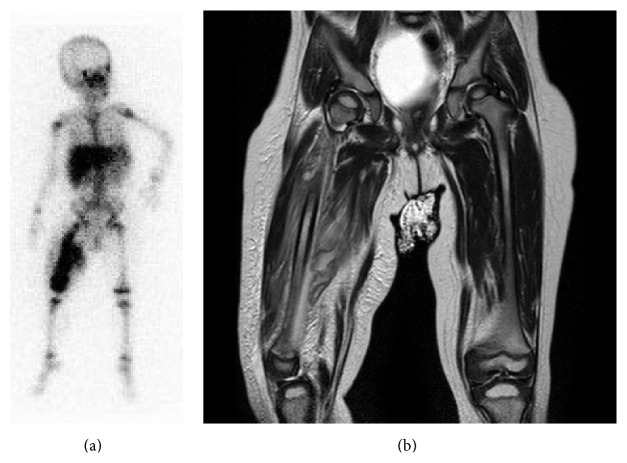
Gallium-67 citrate scintigraphy showing isolated uptake of the right femur (a) and magnetic resonance imaging scans of the right femur showing osteomyelitis with necrotizing fasciitis (T2-weighted image). There are signal changes in the diaphyseal region of the femurs with circumferential soft tissue involvement (b).
